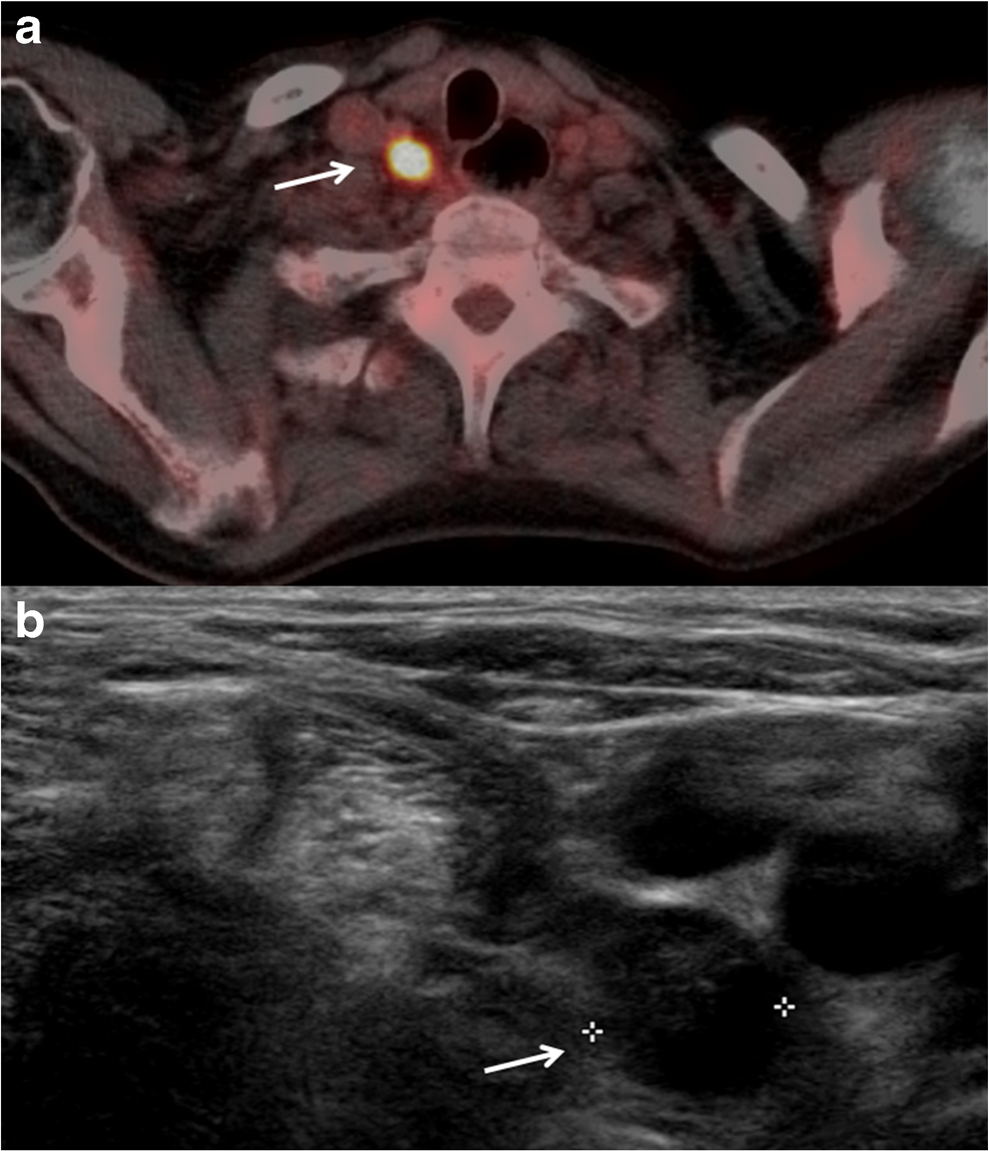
a Fused axial PET/CT image demonstrating a 10-mm FDG-avid cervical lymph node metastasis in station IV (right) during initial staging in a patient with a primary oesophageal squamous cell tumour (arrow). b Cervical ultrasonography image in the same patient, demonstrating a 10-mm cervical round-shaped lymph node metastasis in level IV (right). The cervical lymph node was confirmed malignant with FNA (arrow)
